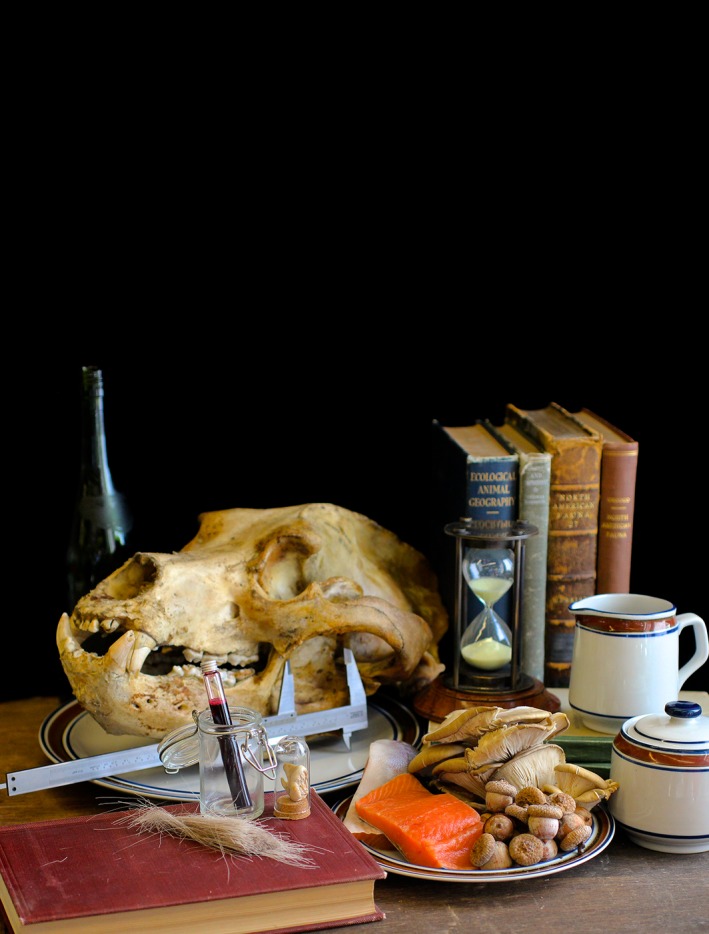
Still life of grizzly bear (*Ursus arctos*) with diet inferred from multiple dietary proxies like gross morphology and isotopes of hair, teeth, and blood. Division of Vertebrate Zoology, YPM MAM 9751. Courtesy of the Peabody Museum of Natural History, Yale University, New Haven, CT, USA.
